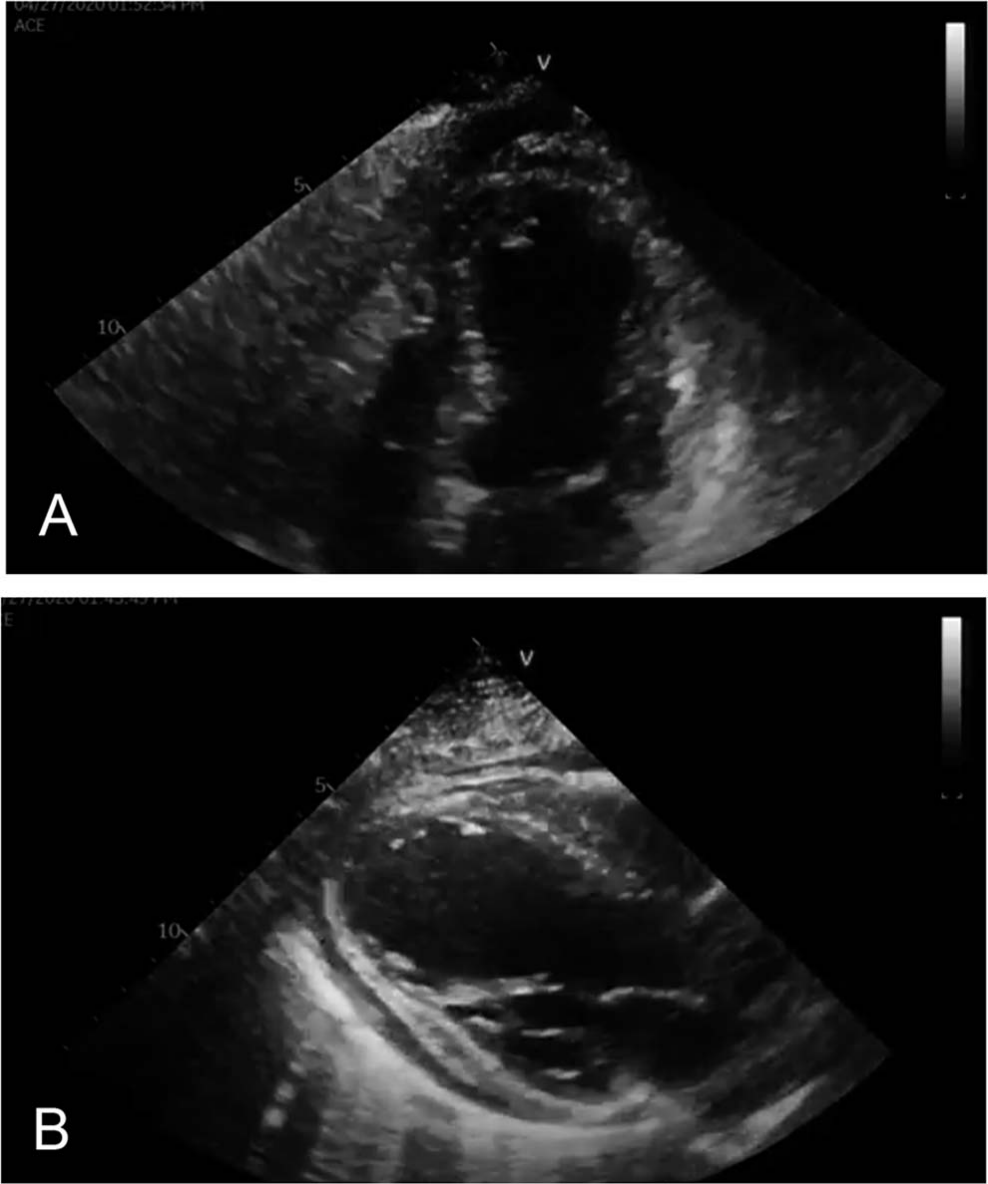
Initial echocardiogram in (A) 4-chamber view shows multiple segmental abnormalities and an ejection fraction of 20%. (B) Parasternal long axis view shows multiple segmental abnormalities, trace effusion, and low ejection fraction of 20%.
